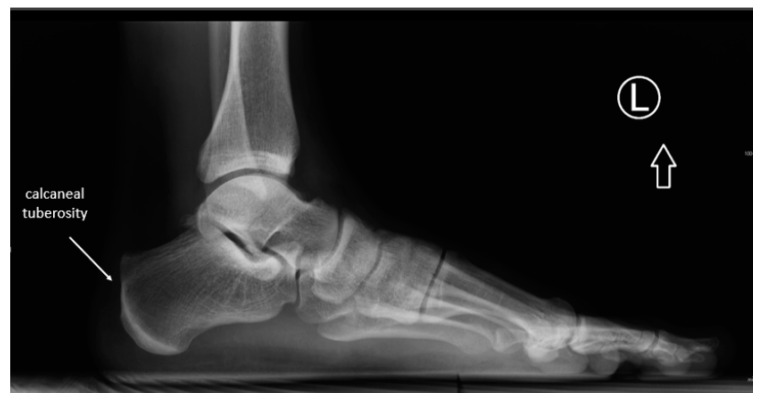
X-ray image of the flat shape of the calcaneal tuberosity.
